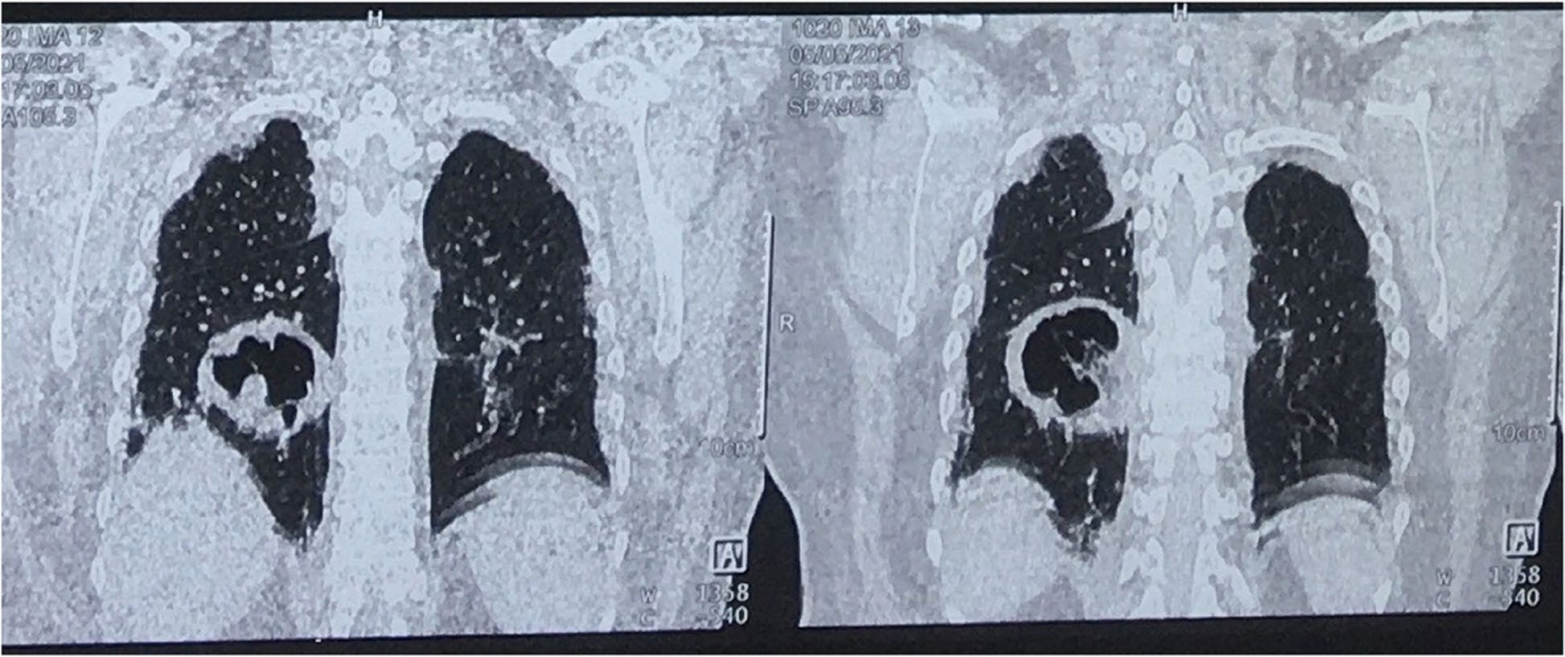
High-resolution CT lung showing multifocal fibrotic changes, honeycombing, and bronchiectasis. Cavitary lesion in the posterior basal segment of the right lower lobe
